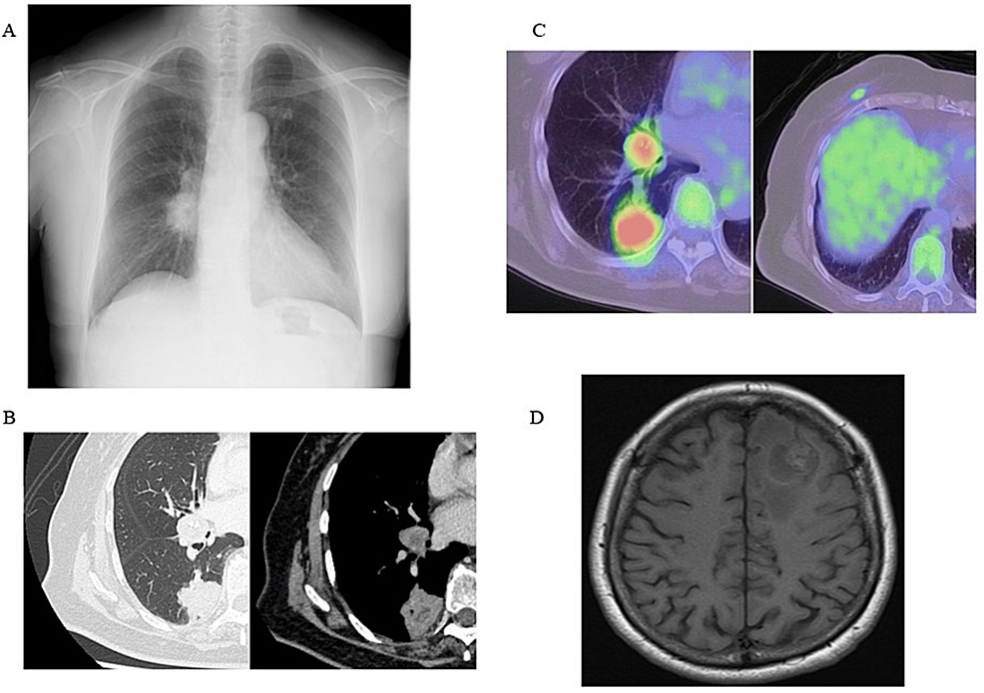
Chest x-ray, computed tomography (CT) findings, positron emission tomography (PET), and magnetic resonance imaging (MRI) of the head before starting Alectinib therapy (A) Chest x-ray shows a mass in the right hilar region. (B) CT scan of the chest shows lung cancer in the right lower lobe and lymph node metastasis in the hilar region. (C) PET scan shows FDG accumulation in right lower lobe lung cancer, lymph node metastasis, and right breast cancer. (D) Head MRI shows a tumor in the left frontal lobe.
